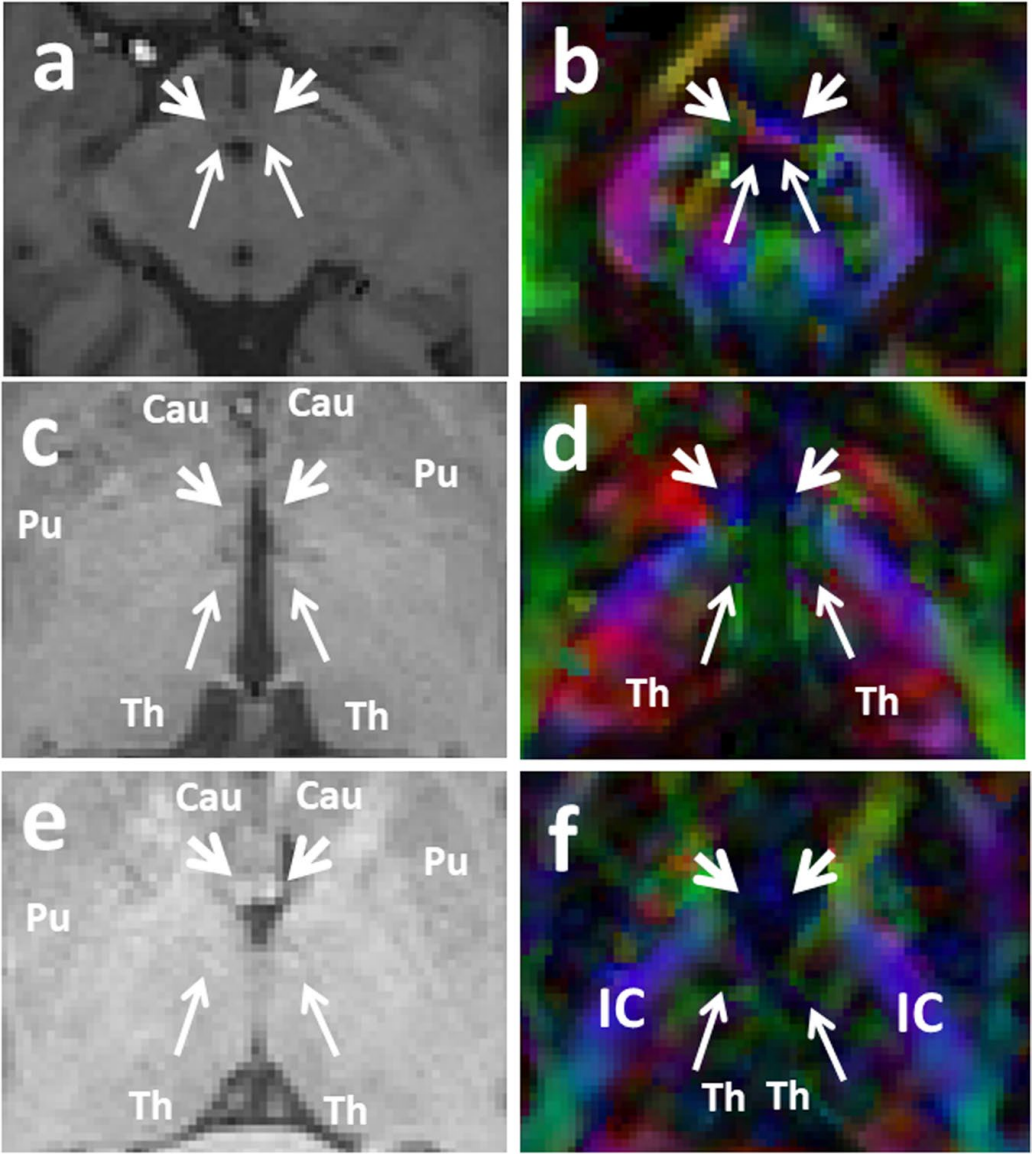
Three successive color-coded DTI map and corresponding T1 weighted MRI sequences in axial planes from caudal to cranial demonstrating the relationship of the postcommissural fibers of the fornix and stria terminalis (pointed by short arrows), with the MTT (pointed by long arrows) as they course parallel to each other. Four bright spots are visible on the T1 weighted images at the level of mammillary bodies and higher levels (a,c,e). The two frontal bright spots represent the forniceal columns. The two dorsal bright spots represent the MTT on both sides (Fig. a,c,e). As it’s visible on Fig. 3a,b, the ascending fibers of the MTT arise from the mammillary bodies (MB), course posteriorly and then cranially behind the poscommissural fibers of the fornix and stria terminalis (pointed by short arrows). The MTT then rises within the anterior thalami (Th) projecting more laterally as its visible on the (e and f). The MTT fibers then terminate in the anterior and medial thalamic nuclei in each side. Cau = caudate head; IC = internal capsule; Pu = putamen; Th = thalamus.
